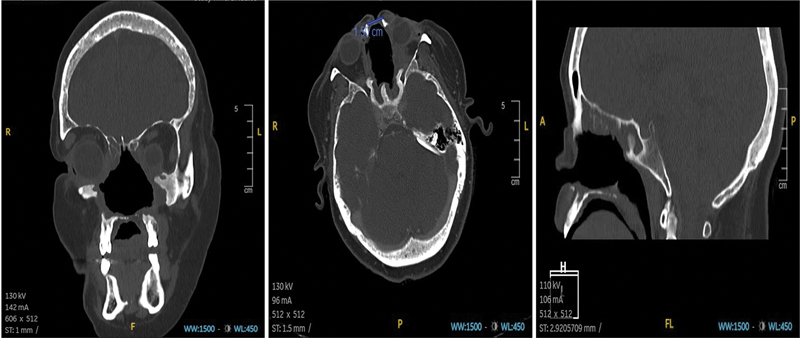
Coronal, axial, and sagittal computed tomography (CT) scans demonstrating the extensive destruction of the osteocartilaginous structures of the midface region.
